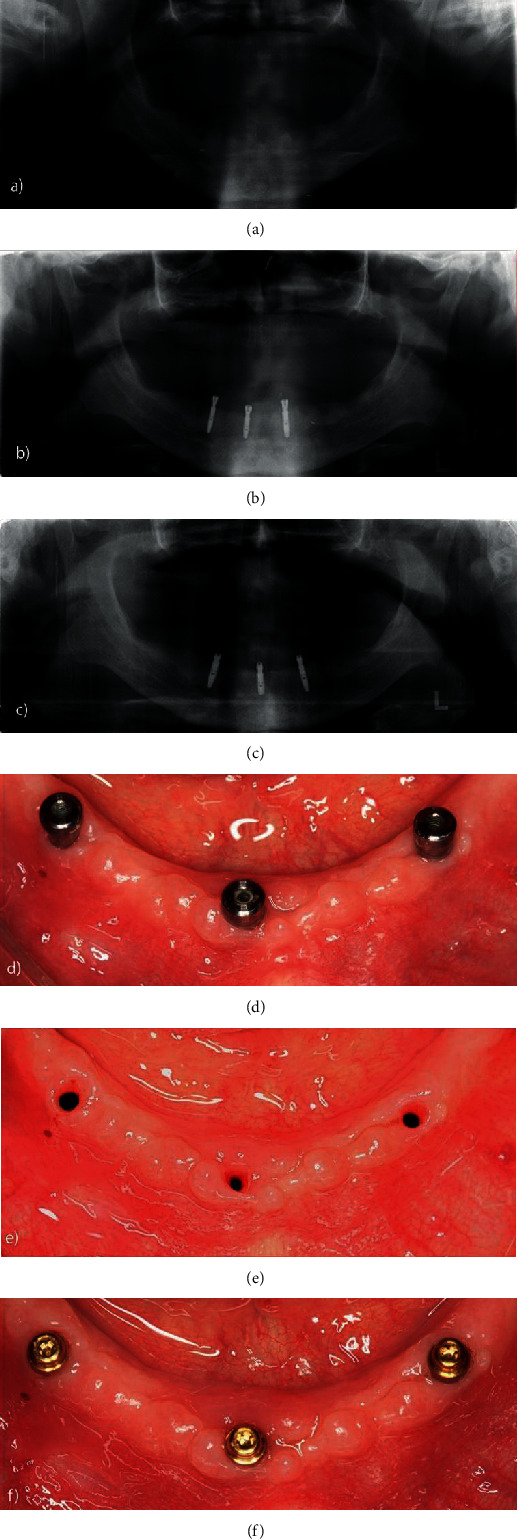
Panoramic X-rays: (a) preoperative, (b) immediately postoperative, and (c) 1 year postoperative; (d) peri-implant healing after 3 months; (e) tissue condition after removal of the healing caps; (f) installation of prosthetic attachment and adaptation of peri-implant tissues around the Equator attachment.
